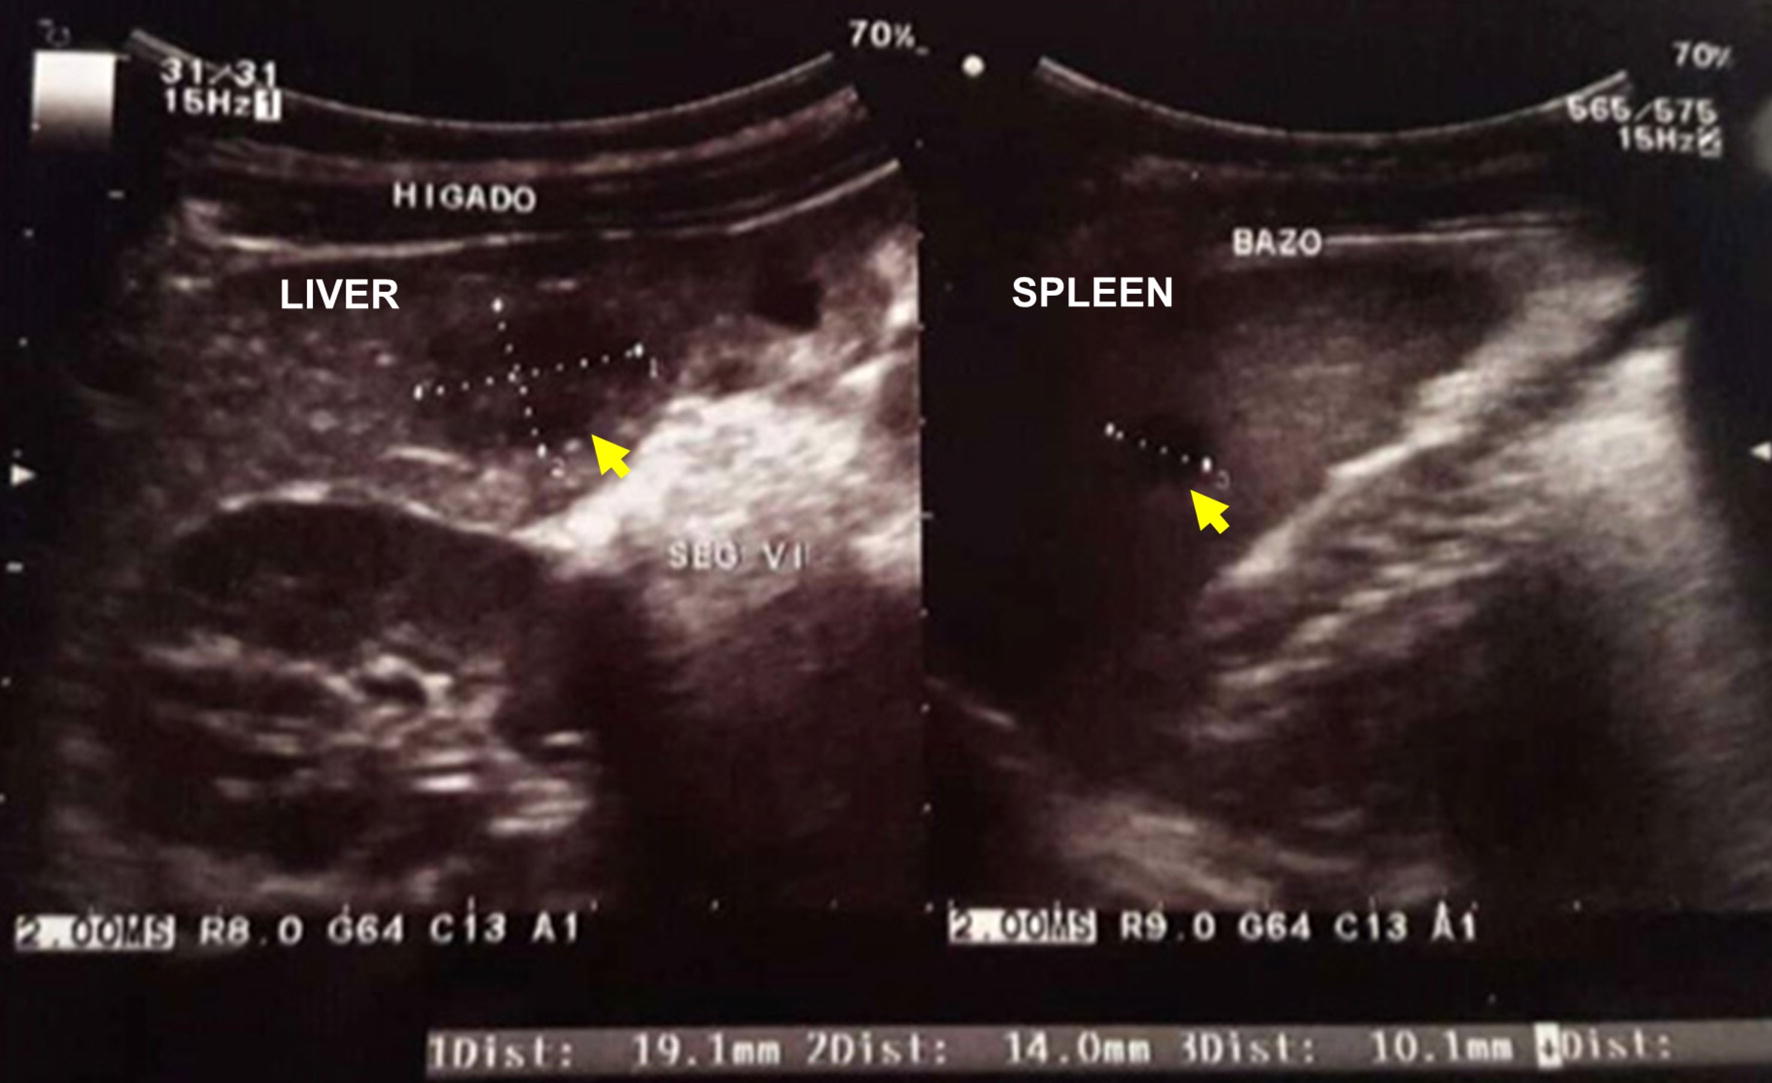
Follow-up ultrasound showing hepatic lesions increased up to 19.1 mm in segments IV and II. Spleen lesions persisted (10.1 mm of diameter) (yellow arrows indicate the lesions)
